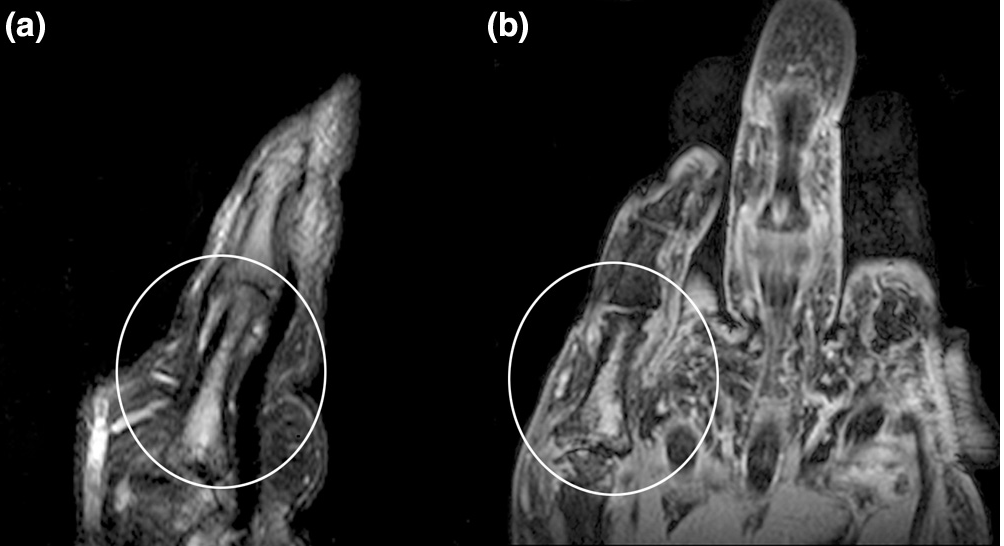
Sagittal T2 weighted fat-saturated (FS) magnetic resonance imaging (MRI) scan of the fifth finger of a patient with non-AM. (a) Diaphyseal bone oedema is shown (circle) and confirmed on (b) coronal post-contrast volumetric interpolated breath-hold examination (VIBE) sequence (circle).
